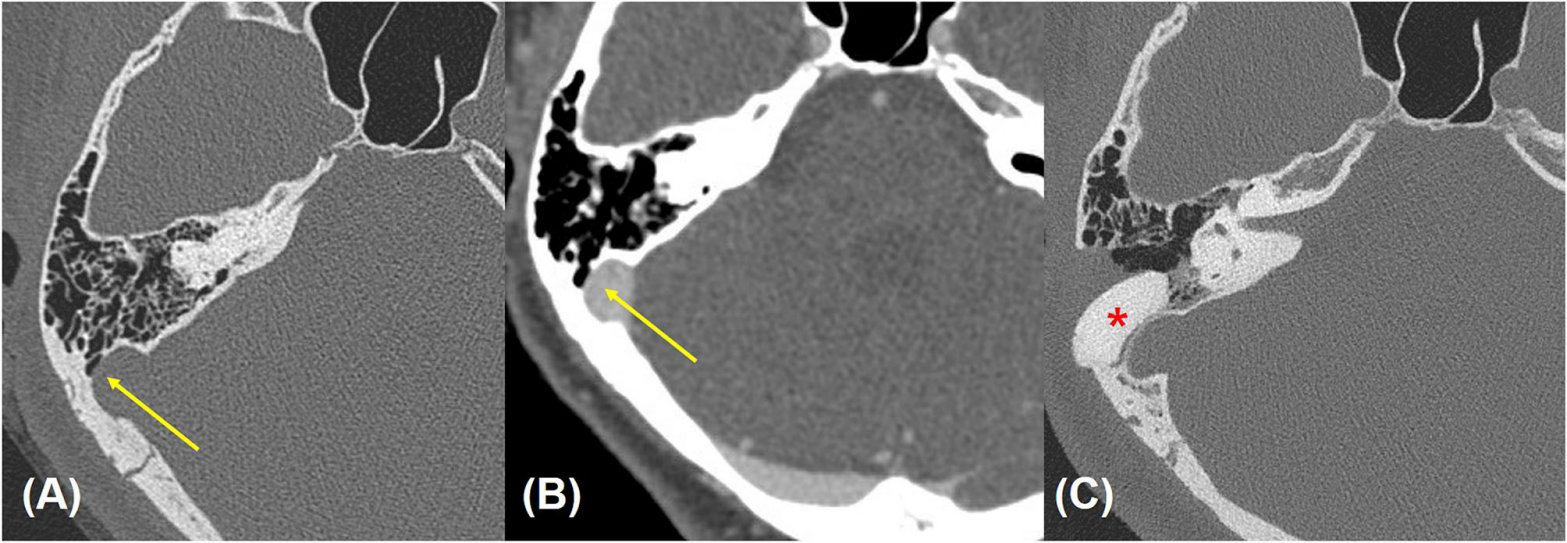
Preoperative (A,B) and postoperative (C) temporal bone computed tomographic angiography (TB-CTA) images of Subject 4. Focal bony dehiscence at the lateral wall of the right transverse-sigmoid junction is observed [yellow arrow points to precontrast (A) and contrast-enhanced (B) images]. (C) Postoperative TB-CTA image showing calcium hydroxyapatite well *in situ* at the previously noted defect site (red asterisk).
